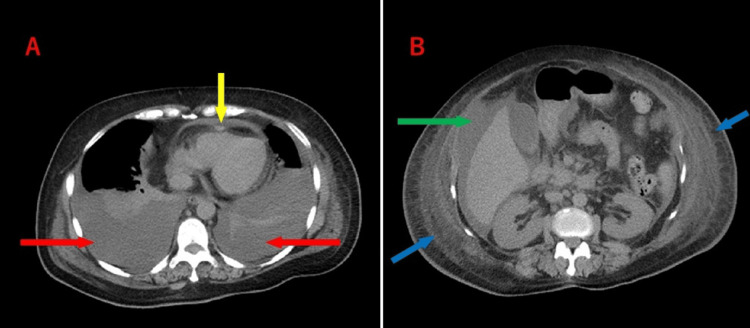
Computed tomography (CT) scan captured at admission to our hospital (A) Chest CT image showing massive bilateral pleural effusion (red arrows) and pericardial effusion (yellow arrow). (B) Abdominal CT image showing ascites (green arrow) and significant edema of subcutaneous tissue (blue arrows).
